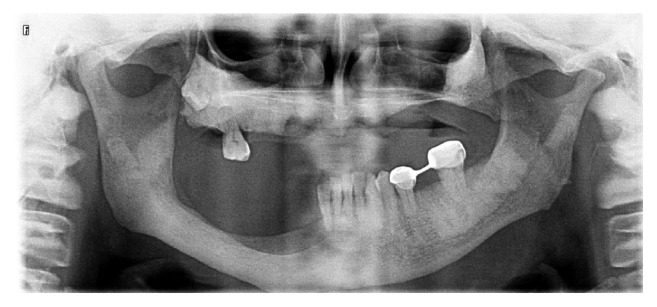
Postoperative panoramic radiography after bony sequestra removed in 2013.
